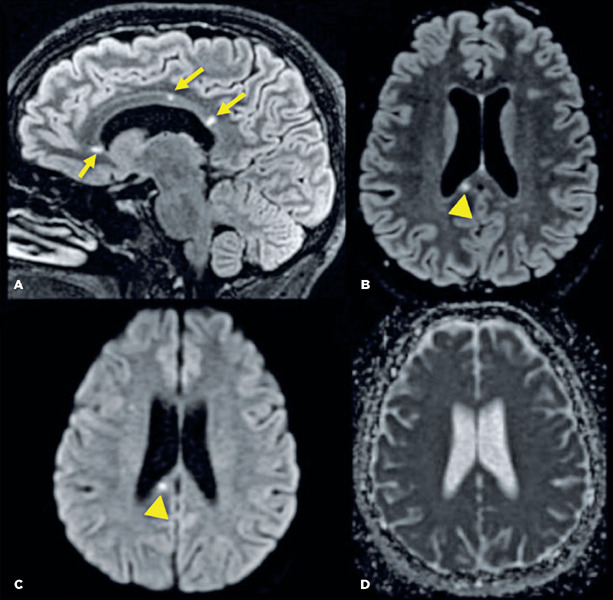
Brain magnetic resonance imaging of a patient with Susac syndrome. Multiple small
